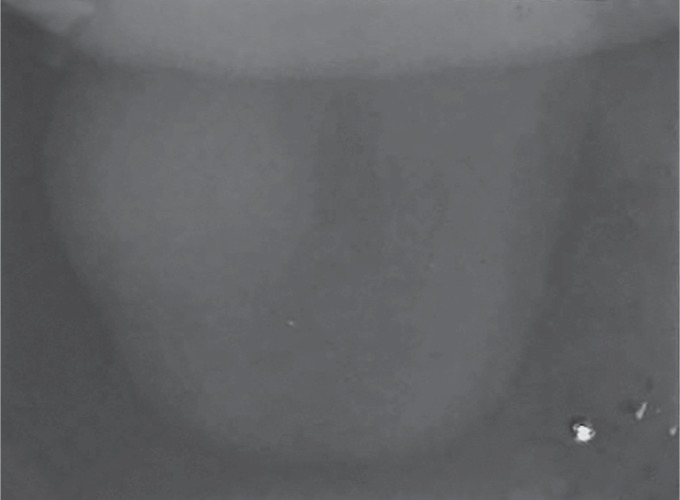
Intraoral periapical radiograph showing the lesion did not reveal any calcifications
